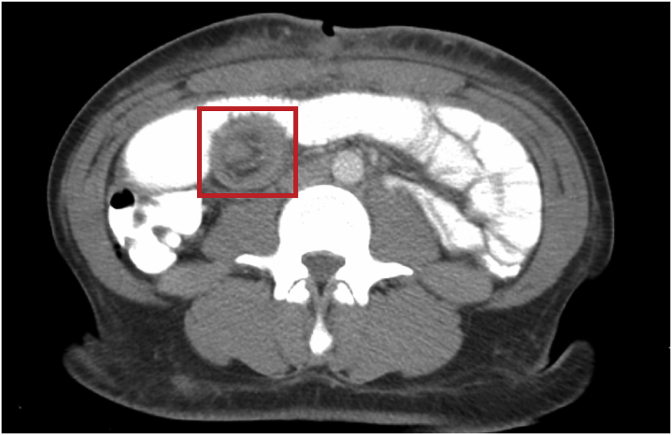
CT scan of the abdomen demonstrating an enteroenteric intussusception in the right-mid abdomen (at the center of the red box). (For interpretation of the references to colour in this figure legend, the reader is referred to the Web version of this article.)
